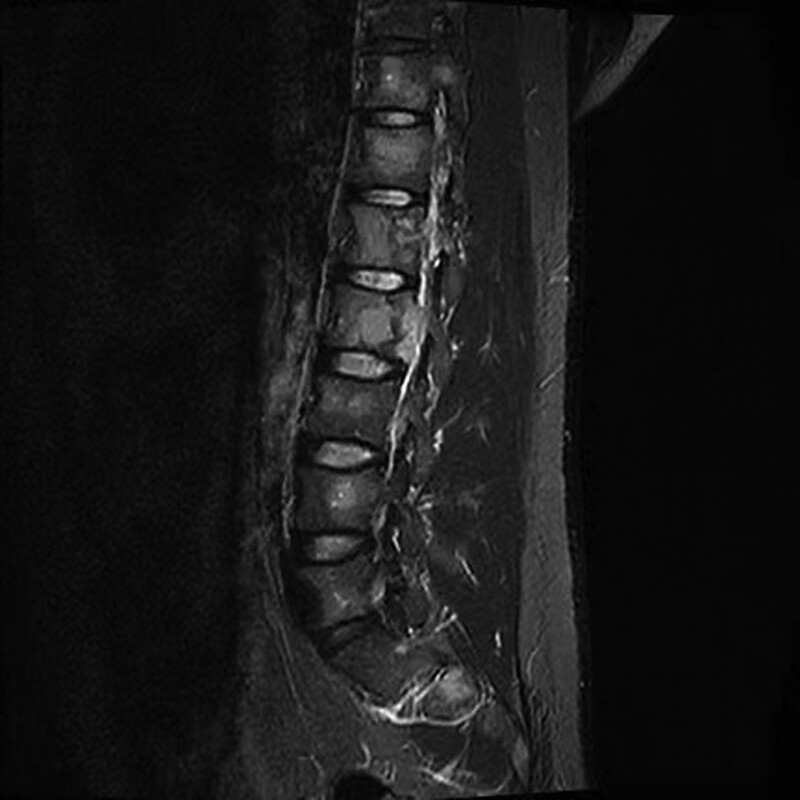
Magnetic resonance imaging revealed abnormal signal shadow of cervical and lumbar vertebrae.
